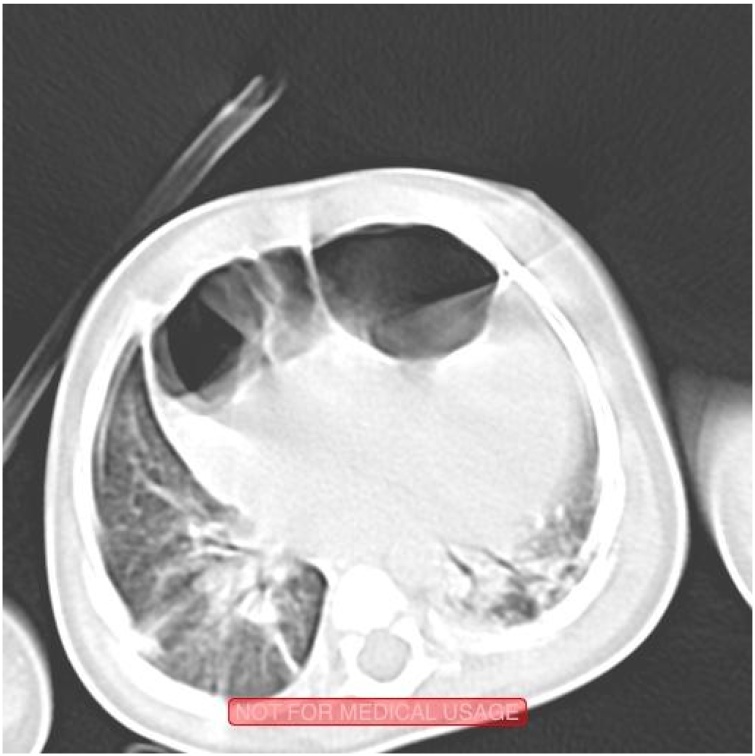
A chest computed tomography scan demonstrating mediastinal loops of bowel.
